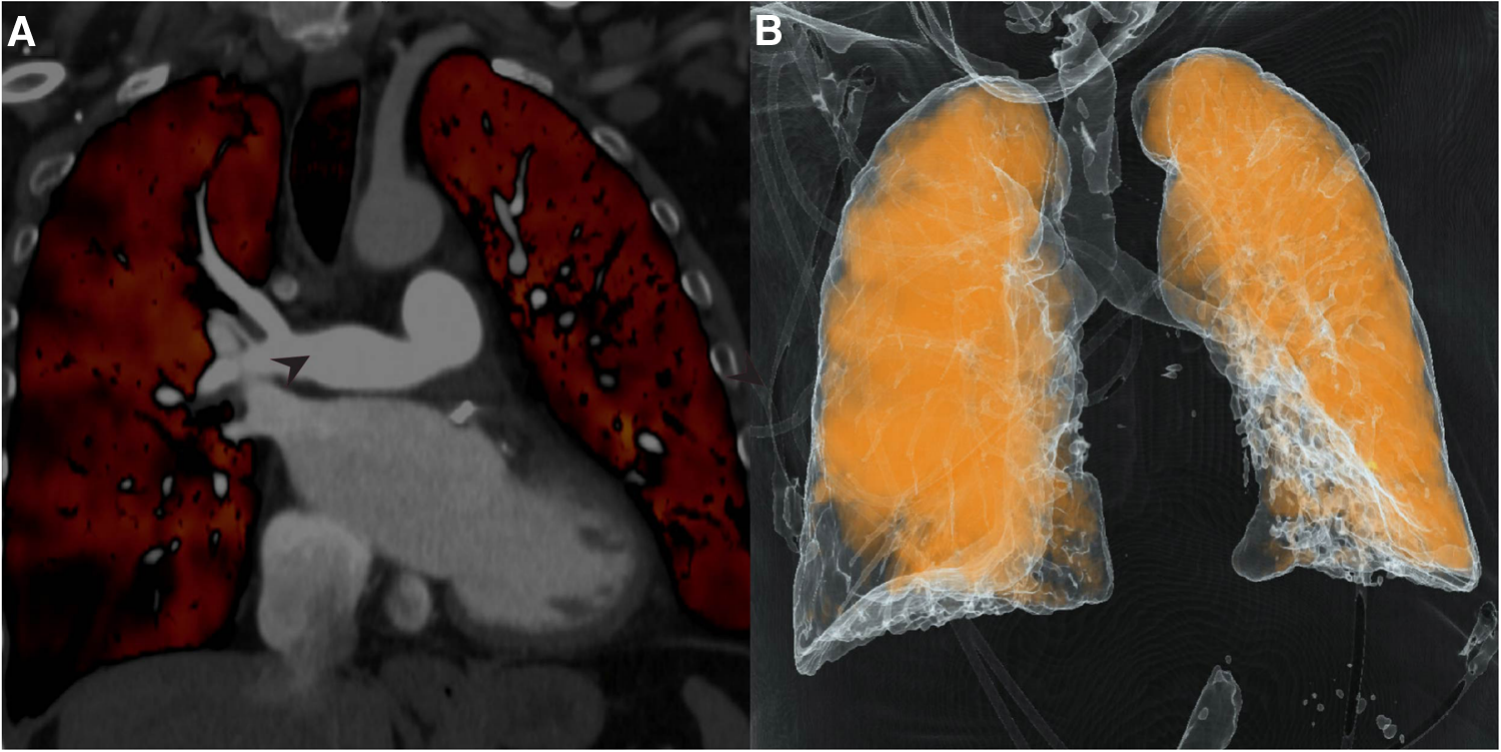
Dual-Energy computed tomography (DECT). (A) Repeat DECT demonstrates patent pulmonary artery post-thromboendarterectomy; (B) Perfusion mapping reveals significantly improved perfusion of right lung.
